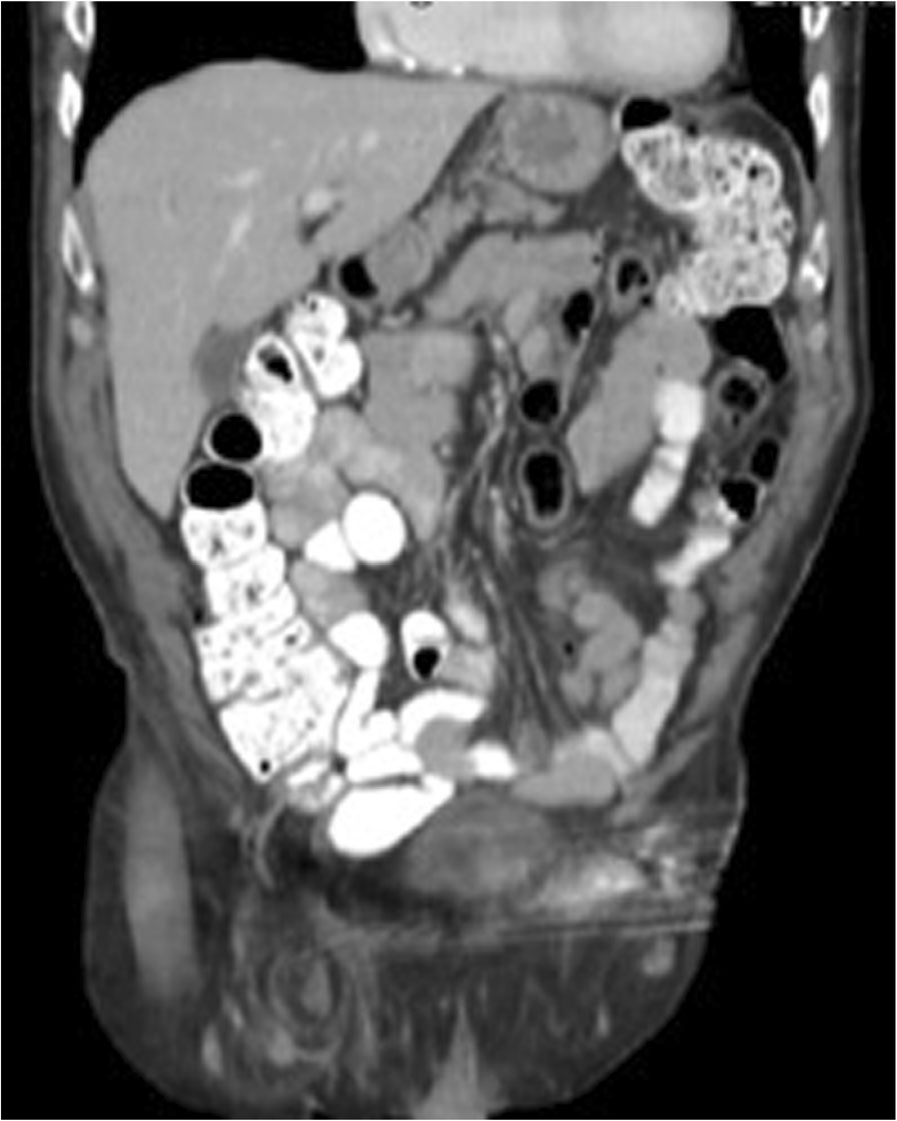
Computed tomography coronal view of described Amyand hernia
